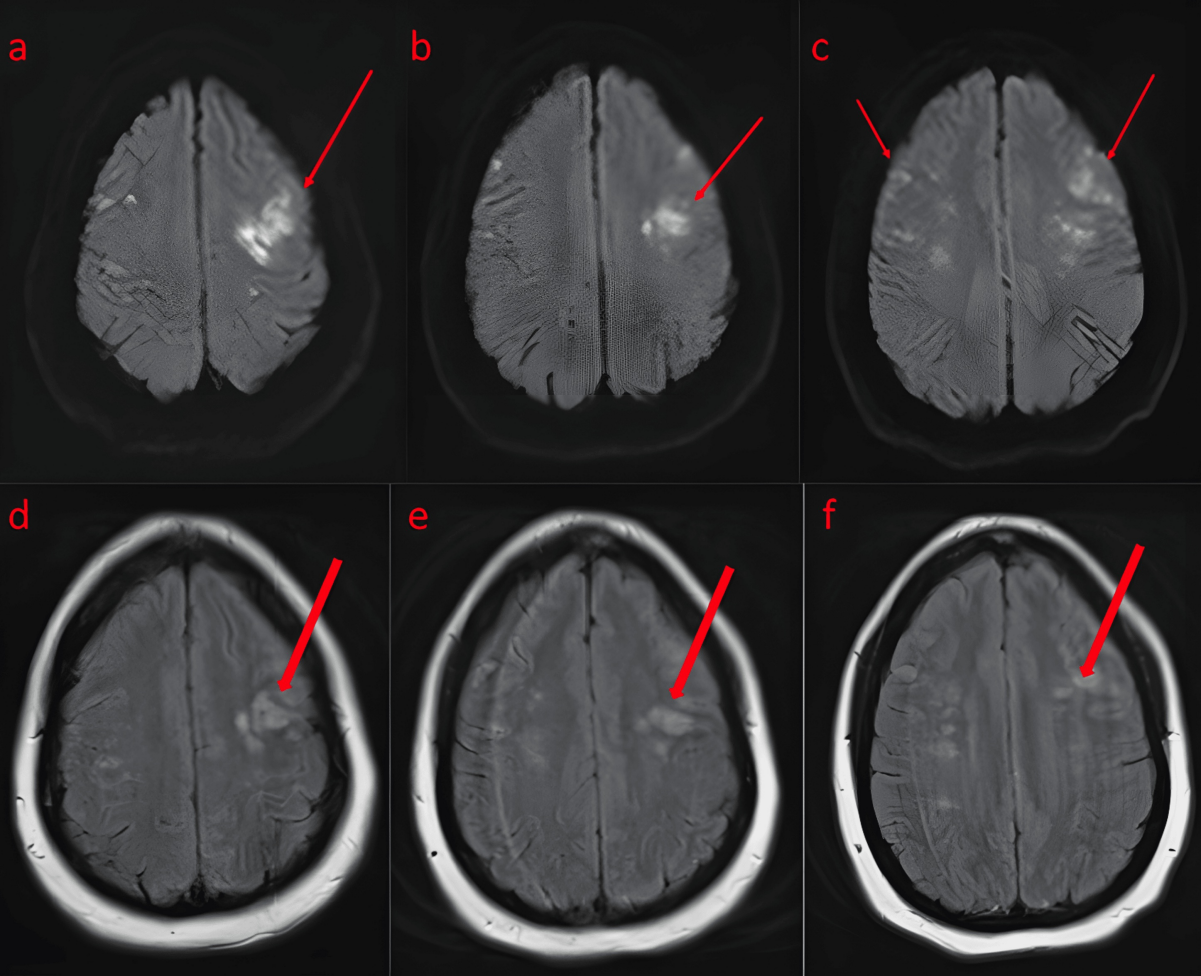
MRI brain with diffusion-weighted imaging sequence showing multiple foci of cortical/subcortical restricted diffusion in the cerebral hemispheres bilaterally, including the temporal lobes (a) and subcortical regions (b). Lesions were found predominantly in the frontal lobes (c) within the watershed distribution, compatible with acute/subacute infarctions. Also shown are FLAIR images (d,e,f), which display acute/subacute infarcts MRI: magnetic resonance imaging
